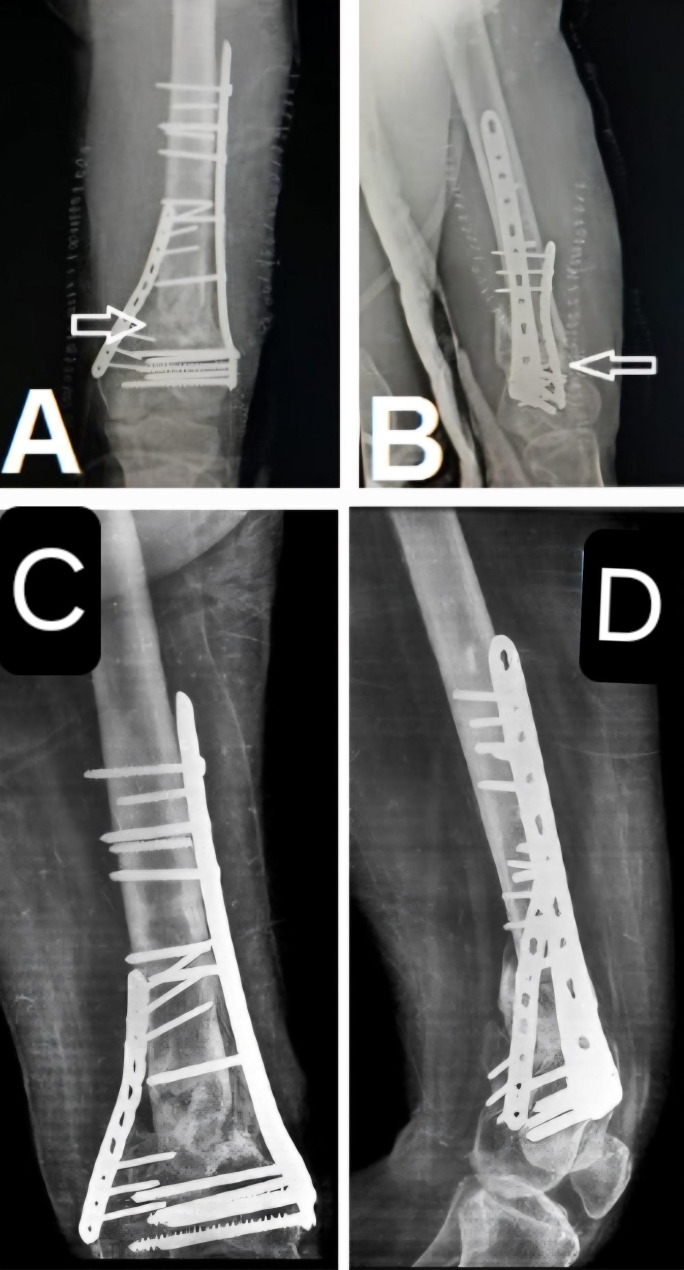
Postoperative X-rays showing the medial femoral condyle corticoperiosteal flap, indicated by the white arrows, in both the anteroposterior (A) and lateral (B) views. At the 6-month follow-up, the X-rays demonstrate fracture union in both views
